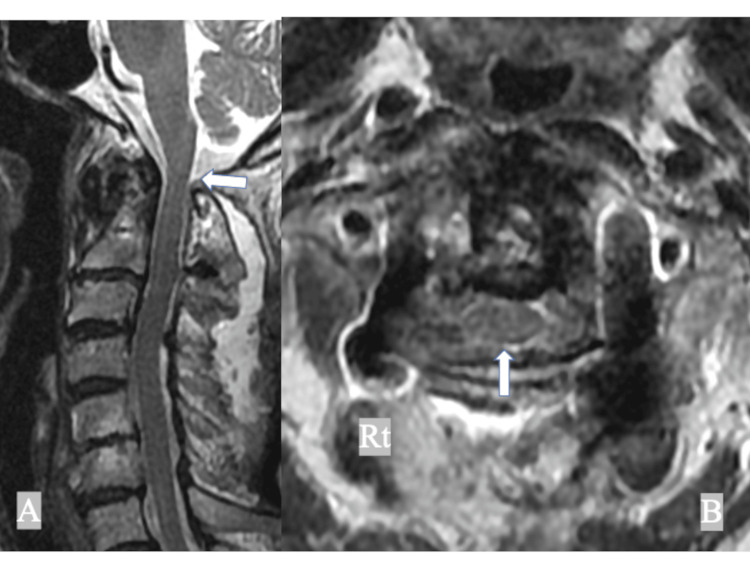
Postoperative MRI of the cervical spine showing (A, B) adequate decompression of the spinal cord at C1/2 (white arrows).
